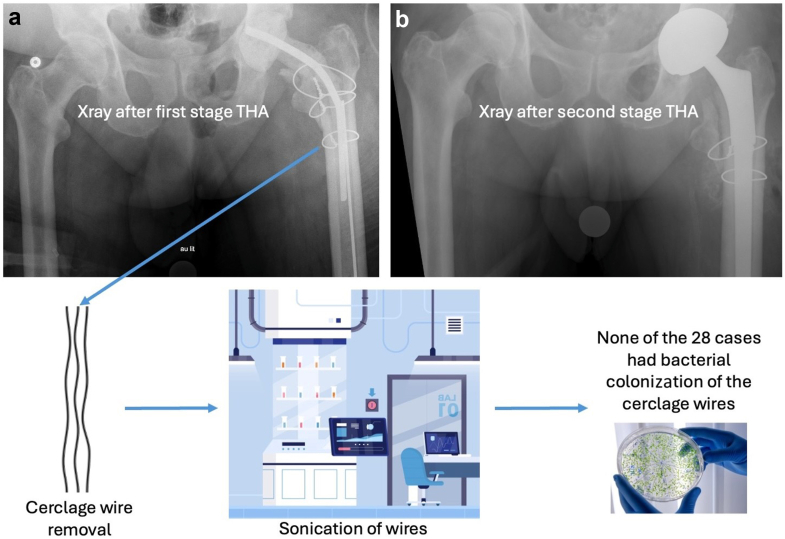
Anteroposterior radiograph of patient 21, a male aged 68 years old, (a) following the first-stage procedure showing the antibiotic spacer and cerclage wires, (b) following the second-stage procedure showing the revision total hip arthroplasty and exchanged cerclage wires.
